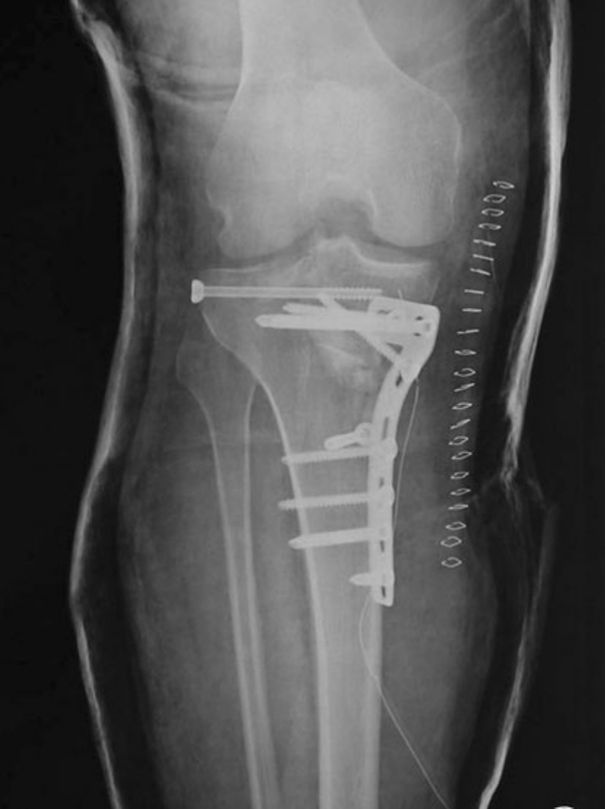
Schatzker type IV fracture, post-op X-rays (AP)
